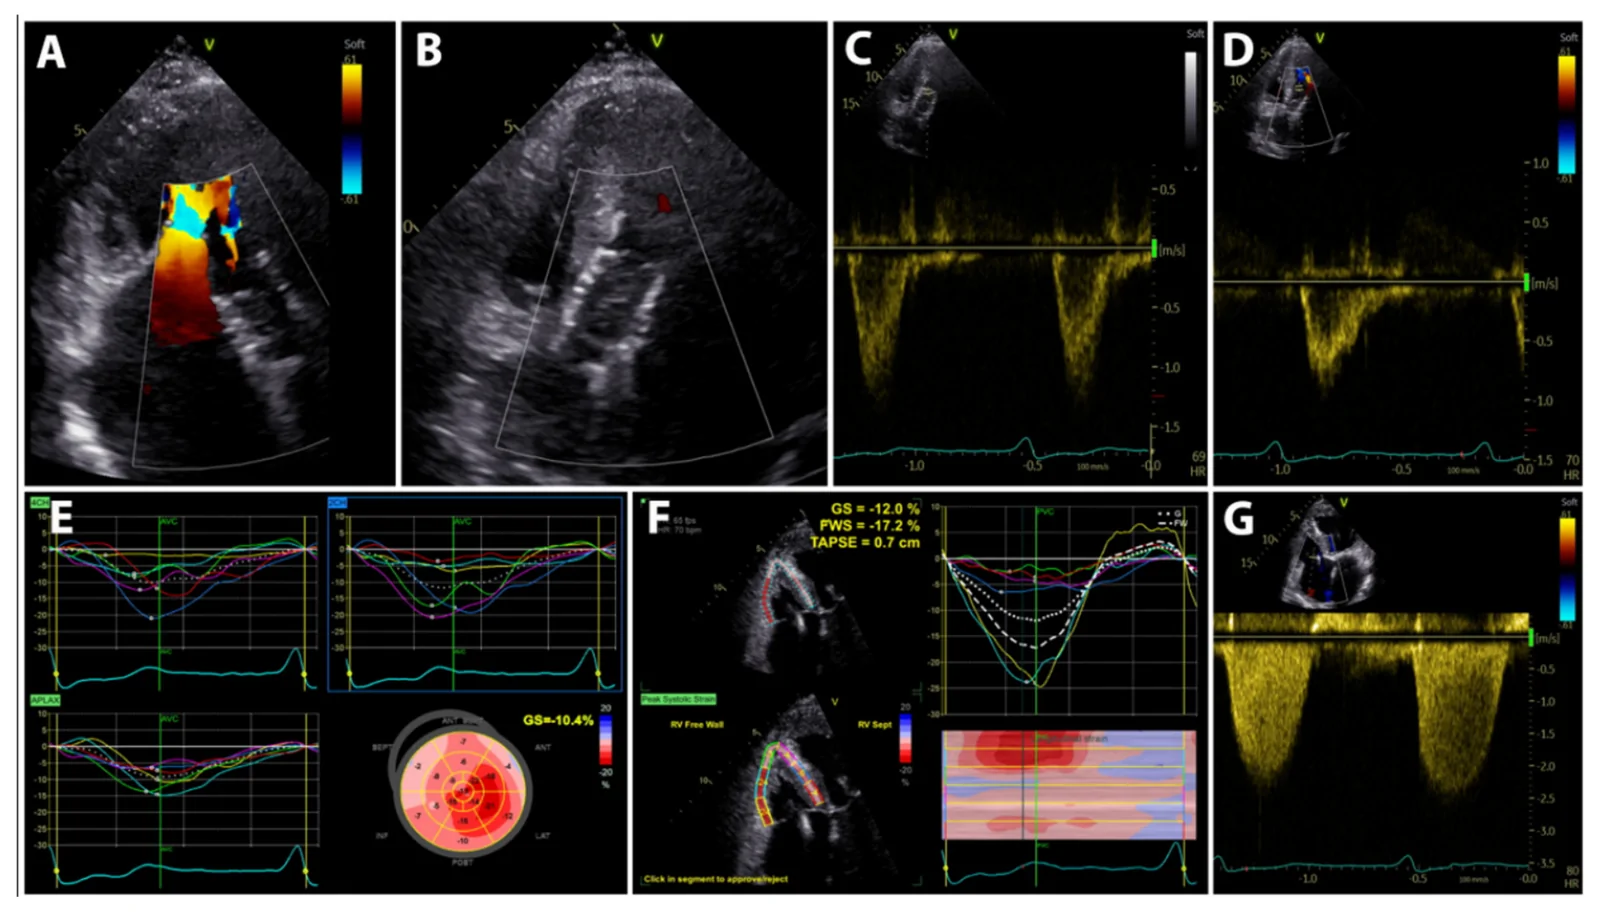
Echocardiographic 1-month follow-up after TAVI. TTE demonstrated a normally functioning Edwards SAPIEN 3 prosthesis with no significant residual aortic regurgitation and normal prosthetic valve hemodynamics (A–D,G). Speckle-tracking echocardiography showed persistent impairment of left ventricular global longitudinal strain and right ventricular longitudinal strain despite marked clinical improvement (E,F).
